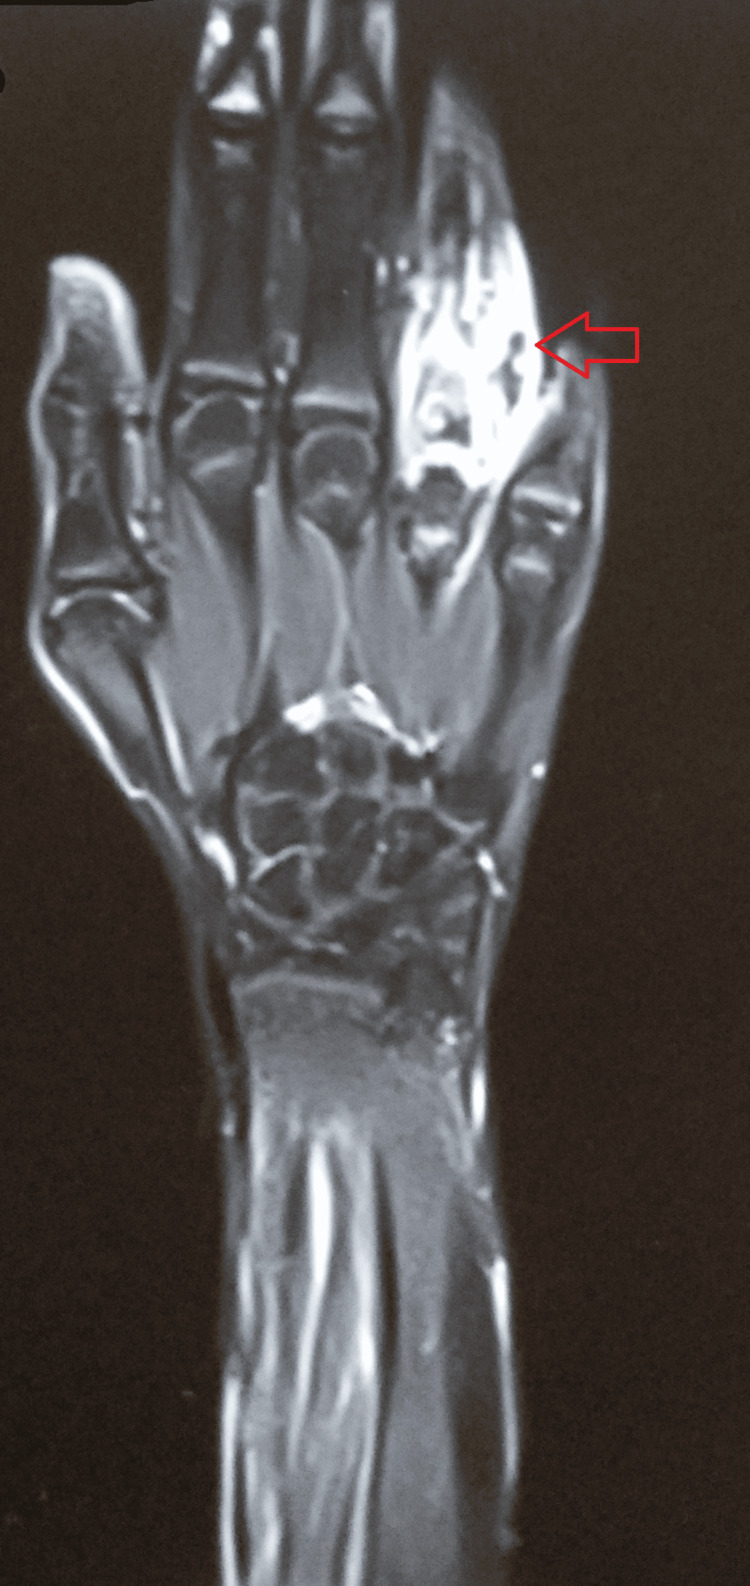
CEMRI of the left upper extremity revealing an altered signal intensity involving the proximal phalanx of the fourth digit. CEMRI: Contrast-enhanced magnetic resonance imaging
